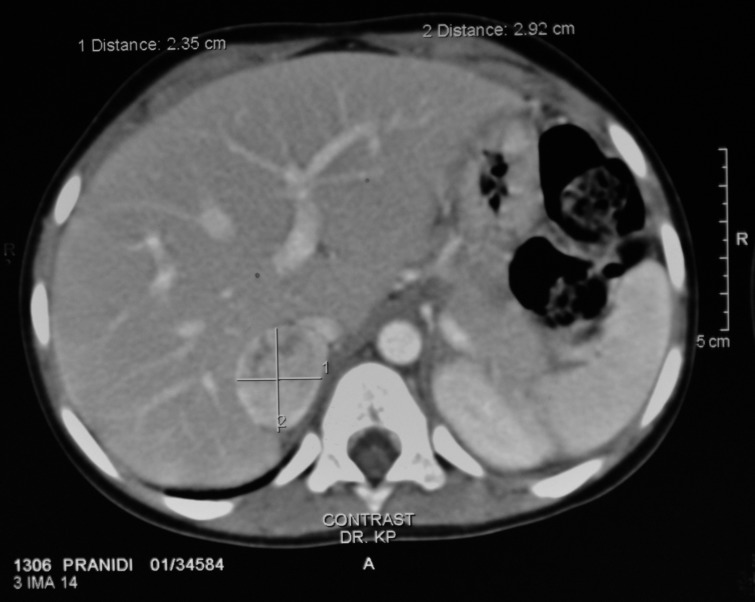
CT abdomen revealing 3.2×2.3×2.9 cm tumour superior to the upper pole of the right kidney.
